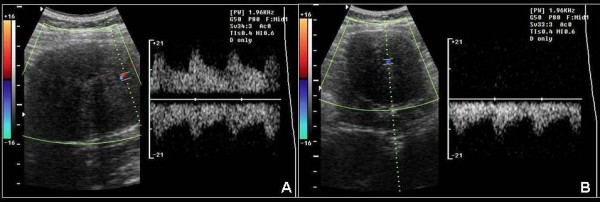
A, B. Color Doppler ultrasound of the abdomen. Color Doppler US detects minimal flow within the mass.
